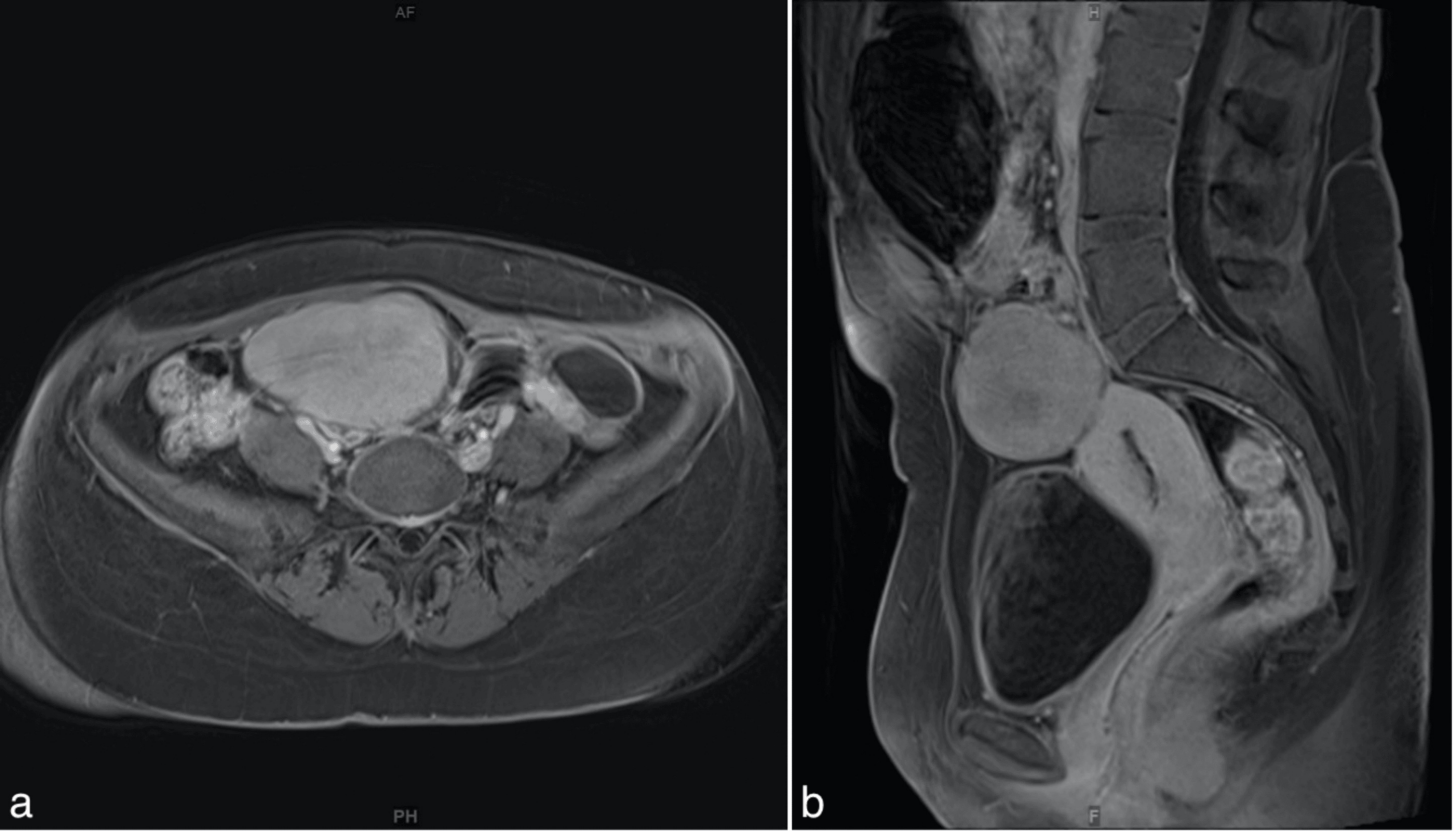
MRI pelvis (1.5 T Magnetom Sola, Siemens Healthineers®) Axial (a) and sagittal (b) fat-suppressed VIBE T1 images in high resolution after IV Gadolinium contrast injection show a homogeneous enhancement of the soft tissue mass. MRI: magnetic resonance imaging
